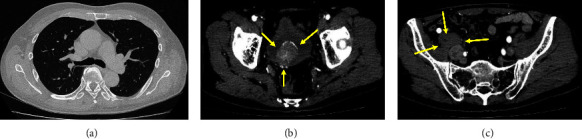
Imaging findings on admission. (a) A chest computed tomography (CT) scan showed no interstitial pneumonia. (b) CT from the abdomen to the pelvis showed a mass lesion protruding from the dorsal wall of the urinary bladder into the lumen, with calcification at the margin (arrows). (c) Swelling thought to be metastasis was observed in the right iliac lymph node (arrows).
